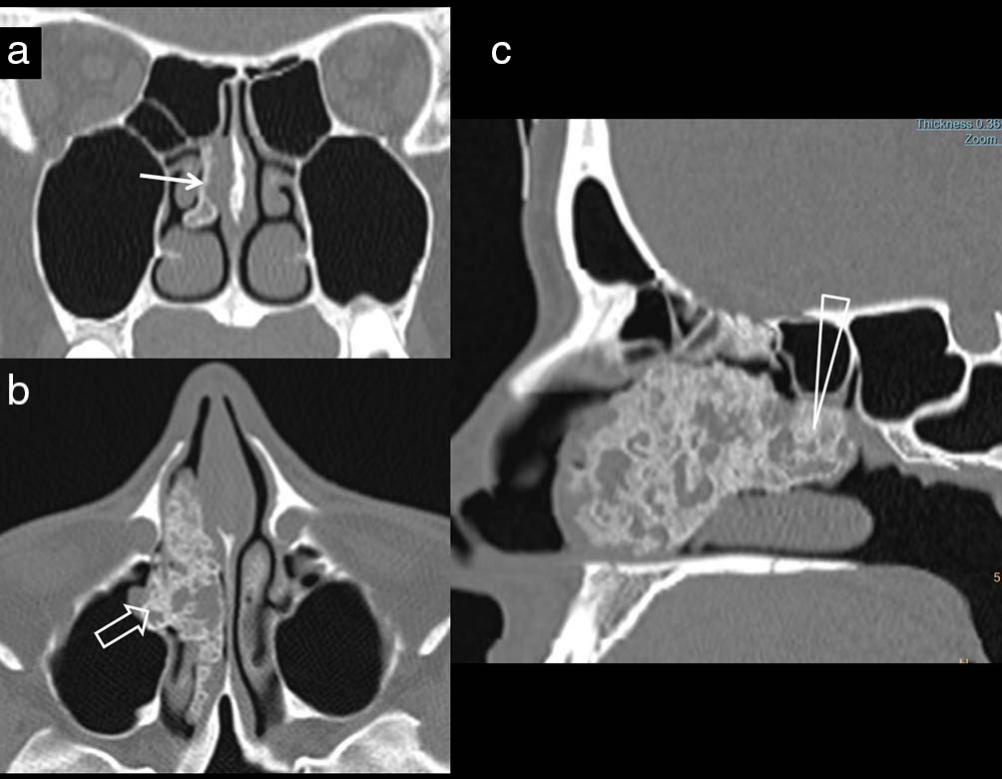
A 31-year-old female patient presenting with nasal block. (a) Non-contrast-enhanced coronal CT images demonstrate a minimally thickened middle turbinate (arrow). (b) The axial image demonstrates extensive areas of heterogeneous calcification in the mid-nasal cavity (open arrow), extending anteriorly to the right nasal vestibule. (c) Parasagittal CT reconstruction demonstrates the lesion extending to the basal lamella (triangle).
